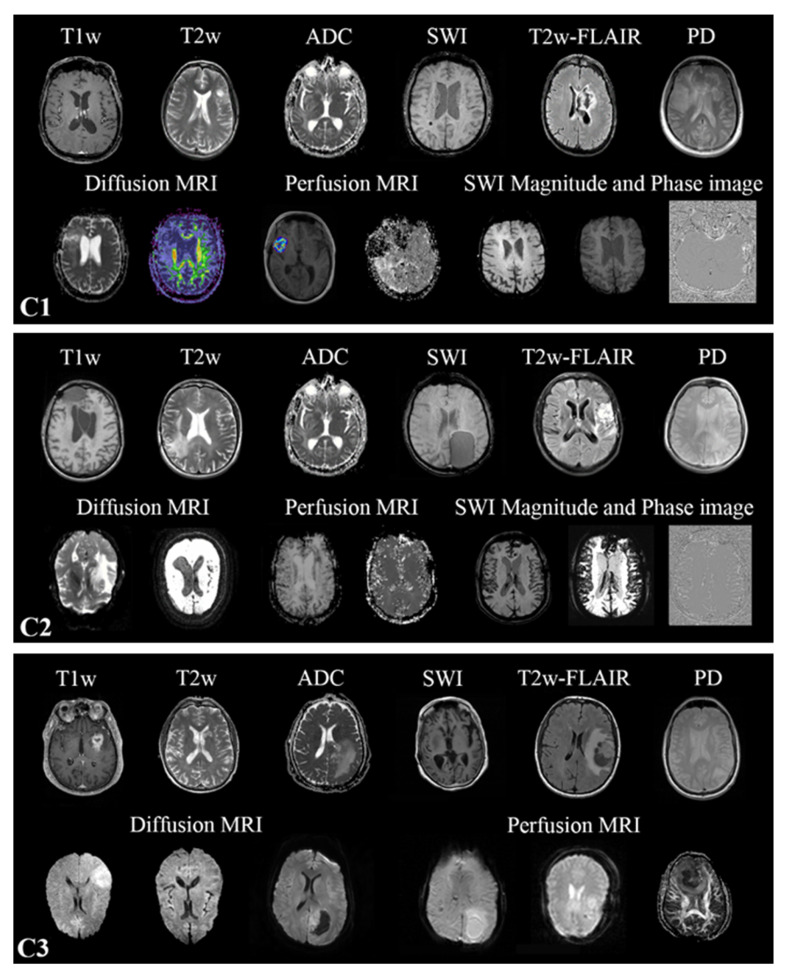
Sample images of the different MR images present in the three datasets C1–C3.
